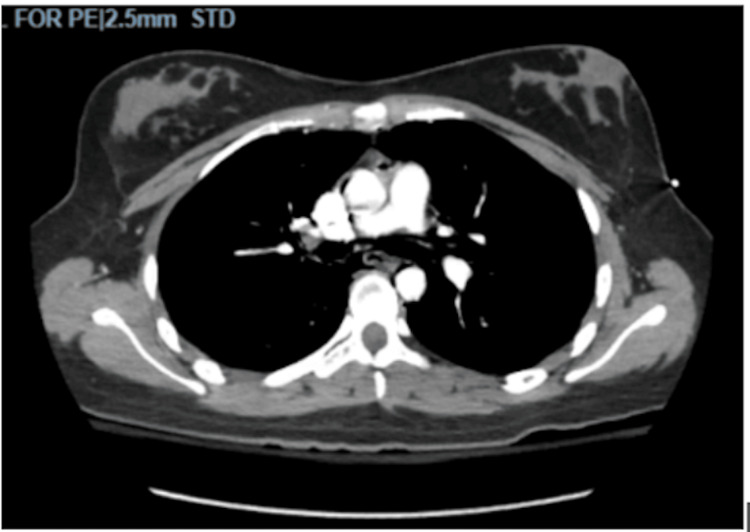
Findings of CT angiography of the chest (sagittal view). There is diffuse subcutaneous emphysema in the neck and pneumomediastinum. There is diffuse esophageal wall thickening, and esophageal perforation cannot be excluded. There is no significant mediastinal or hilar adenopathy. No pulmonary emboli, pulmonary infiltrates, pleural effusion, or pneumothorax can be identified.
